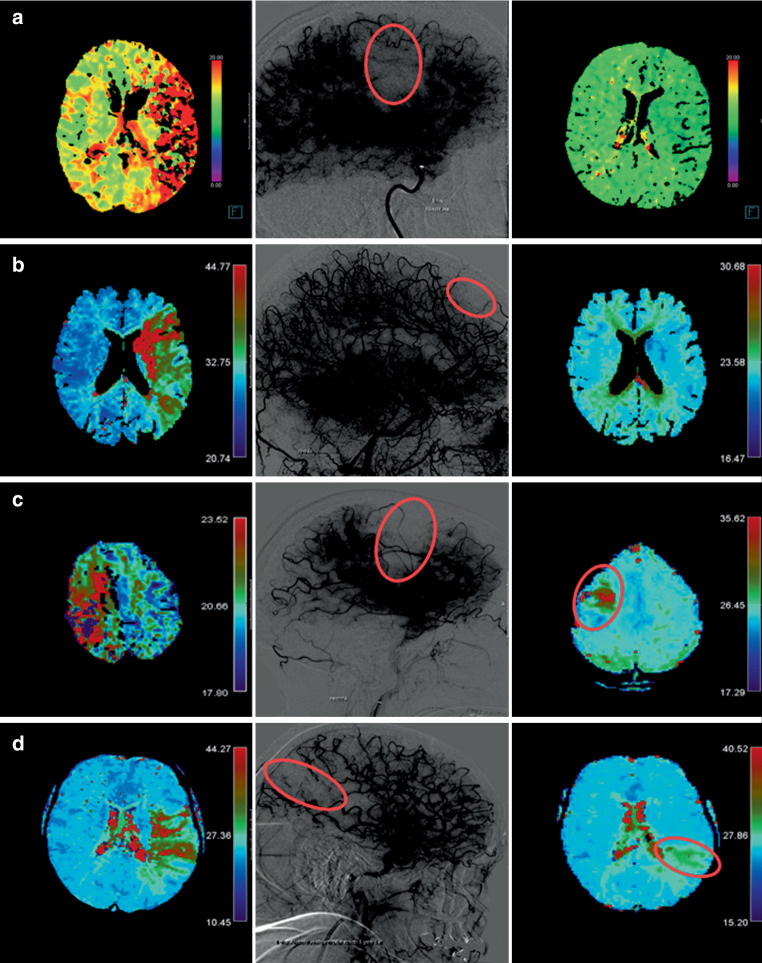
Delayed reperfusion (DR) and persistent perfusion deficit (PPD) on perfusion follow-up imaging. Final angiography runs (*middle panel*) are displayed with high contrast in order to emphasize the capillary phase deficits. TTP and Tmax perfusion imaging maps were evaluated on admission (*left panel*) and follow up (*right panel*) examinations due to their high sensitivity rates (Olea Sphere v2.3; Olea Medical & syngo.via, Siemens). a Patient with a left side M1 occlusion with DR on CT follow-up after extended thrombolysis in cerebral infarction (eTICI) 2b50 reperfusion. b Patient with a left side ICA occlusion with DR on MRI follow-up after eTICI 2c reperfusion. c Patient with a right side M2 occlusion with PPD on MRI follow-up after eTICI 2b50 reperfusion. d Patient with a left side M1 occlusion with PPD on MRI follow-up after eTICI 2b50 reperfusion
